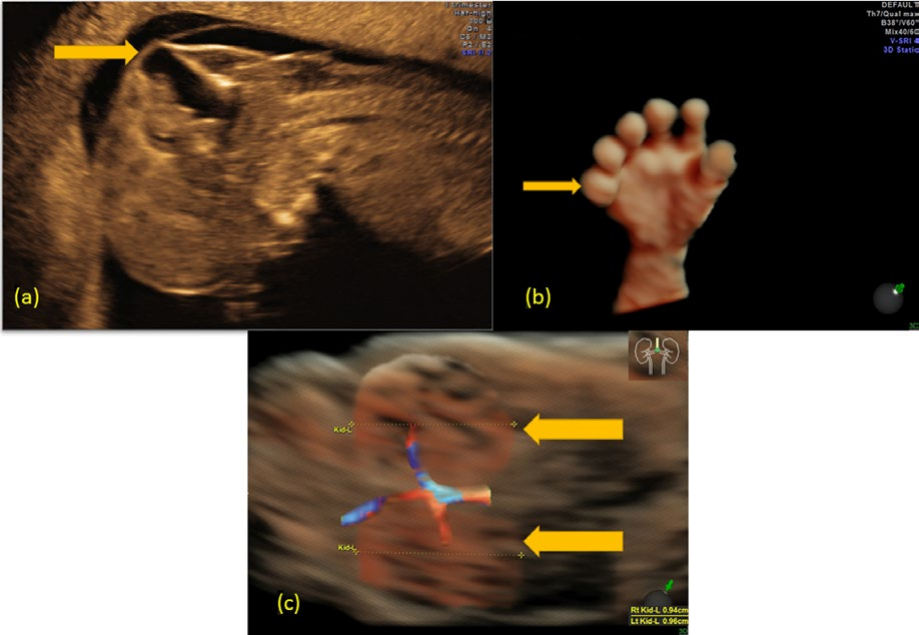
(a) Occipital encephalocele in two‐dimensional high‐resolution transvaginal ultrasound: midsagittal view shows the large occipital bone defect through which the meninges and cerebral parenchyma have migrated. (b) Postaxial polydactyly of one hand in three‐dimensional (3D) high‐resolution transvaginal ultrasound in surface mode demonstrating postaxial view of the extra digit (six digits). (c) Cystic renal dysplasia in 3D high‐resolution transvaginal ultrasound in constructed glass body mode: coronal view shows enlarged hyperechoic kidneys leading to distention of the abdomen
